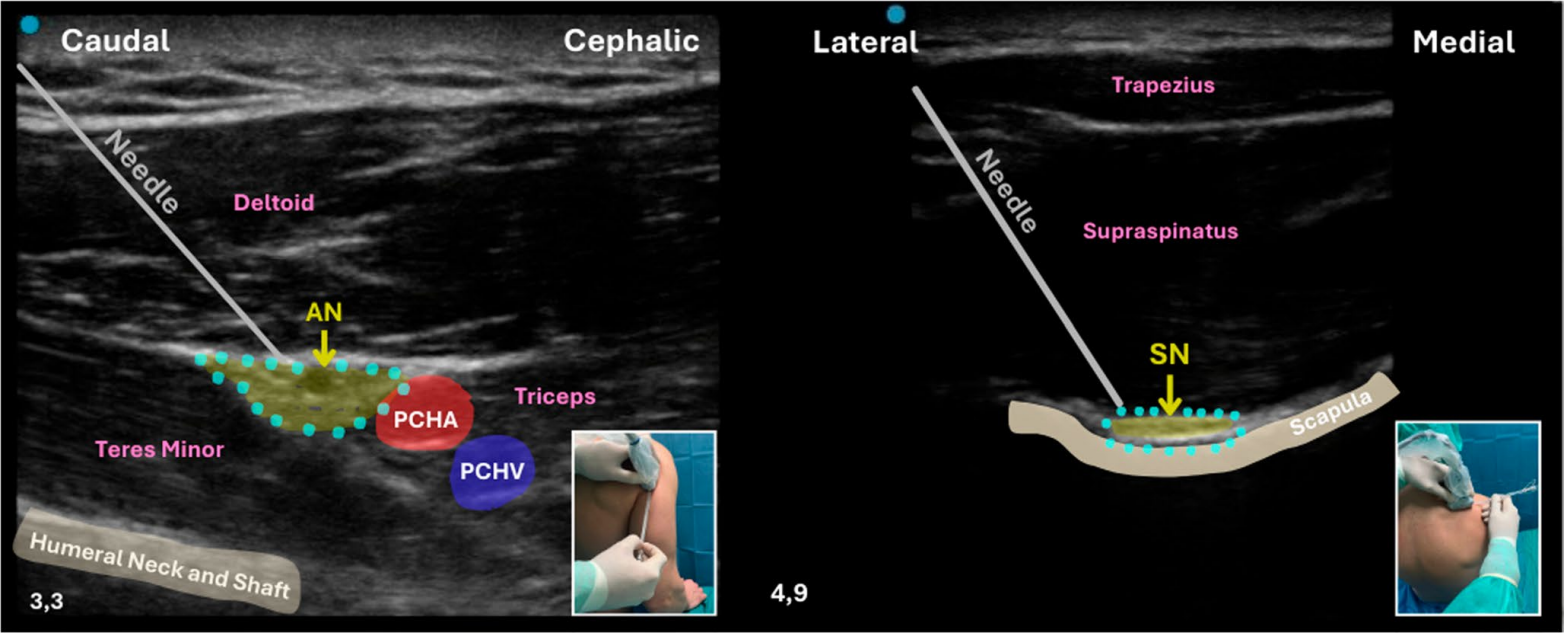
Ultrasound-guided combined AxNB + SSNB. On the left, axillary nerve block performed via a posterior approach, with an in-plane needle trajectory directed from caudal to cranial. The axillary nerve (AN) is visualized near the posterior circumflex humeral artery (PCHA) and vein (PCHV), between the deltoid and teres minor muscles. On the right, suprascapular nerve block performed through a lateral-to-medial in-plane needle approach at the supraspinous fossa, targeting the suprascapular nerve (SN) beneath the supraspinatus muscle. In both panels, the blue dots indicate the target area for local anesthetic injection. Insets show the corresponding transducer and needle positioning. Abbreviations: AN – Axillary Nerve; SN – Suprascapular Nerve; PCHA – Posterior Circumflex Humeral Artery; PCHV – Posterior Circumflex Humeral Vein
